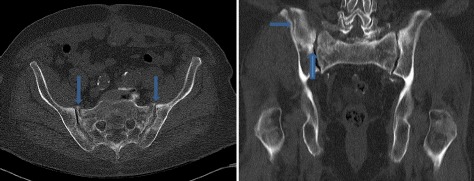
Fracture of the right iliac crest and pelvic ring fracture (computed tomography scan) (*arrows*)
